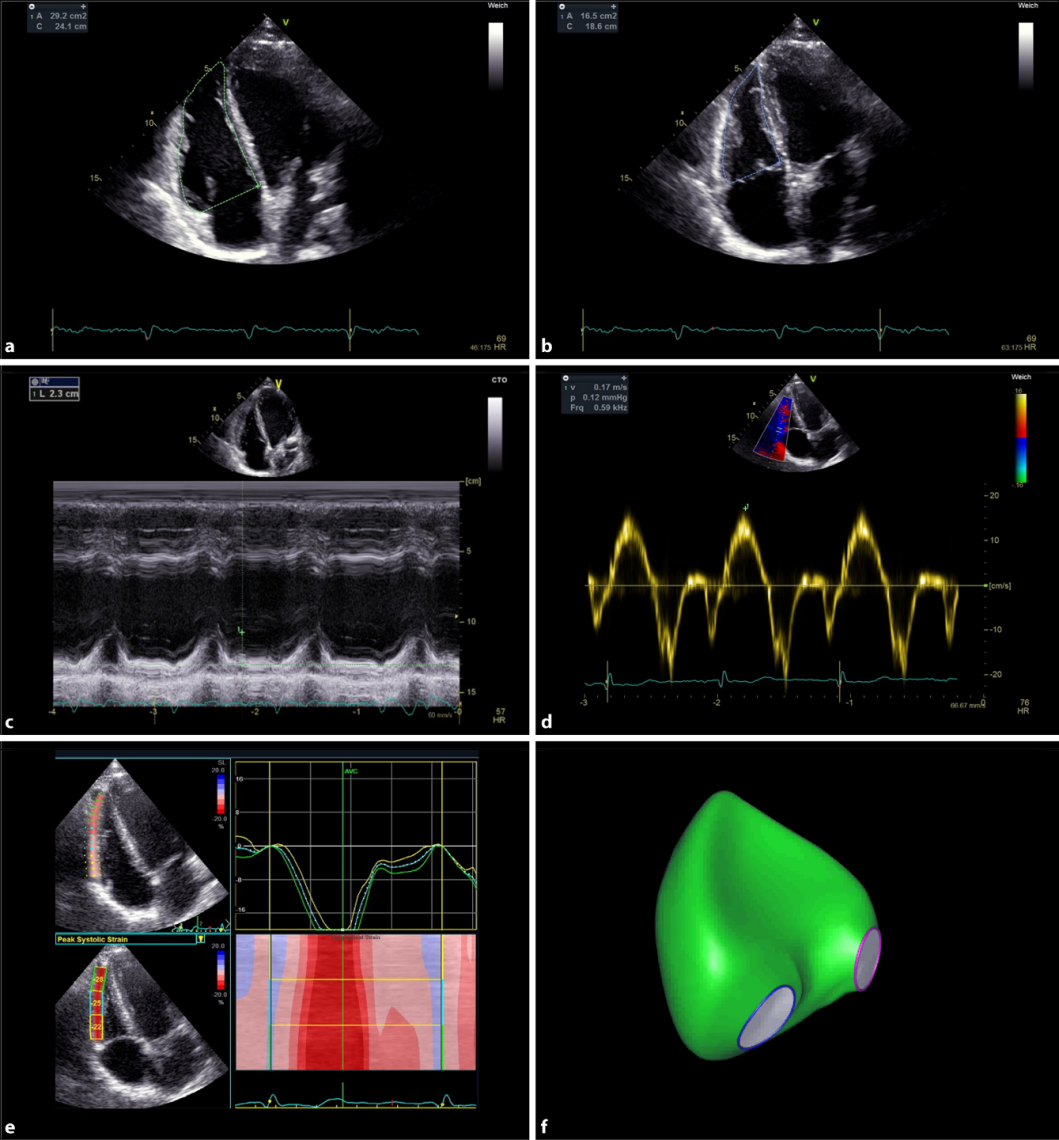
Evaluation of right ventricular function: RV end-diastolic (panel a) and end-systolic (panel b) area for calculation of right ventricular fractional area change. Tricuspid annular plane systolic excursion (TAPSE) (panel c) and Tissue Doppler of the free lateral wall (S’) (panel d), longitudinal strain of the free lateral wall of the right ventricle (panel e), and transthoracic 3D reconstruction of the right ventricle (panel f)
